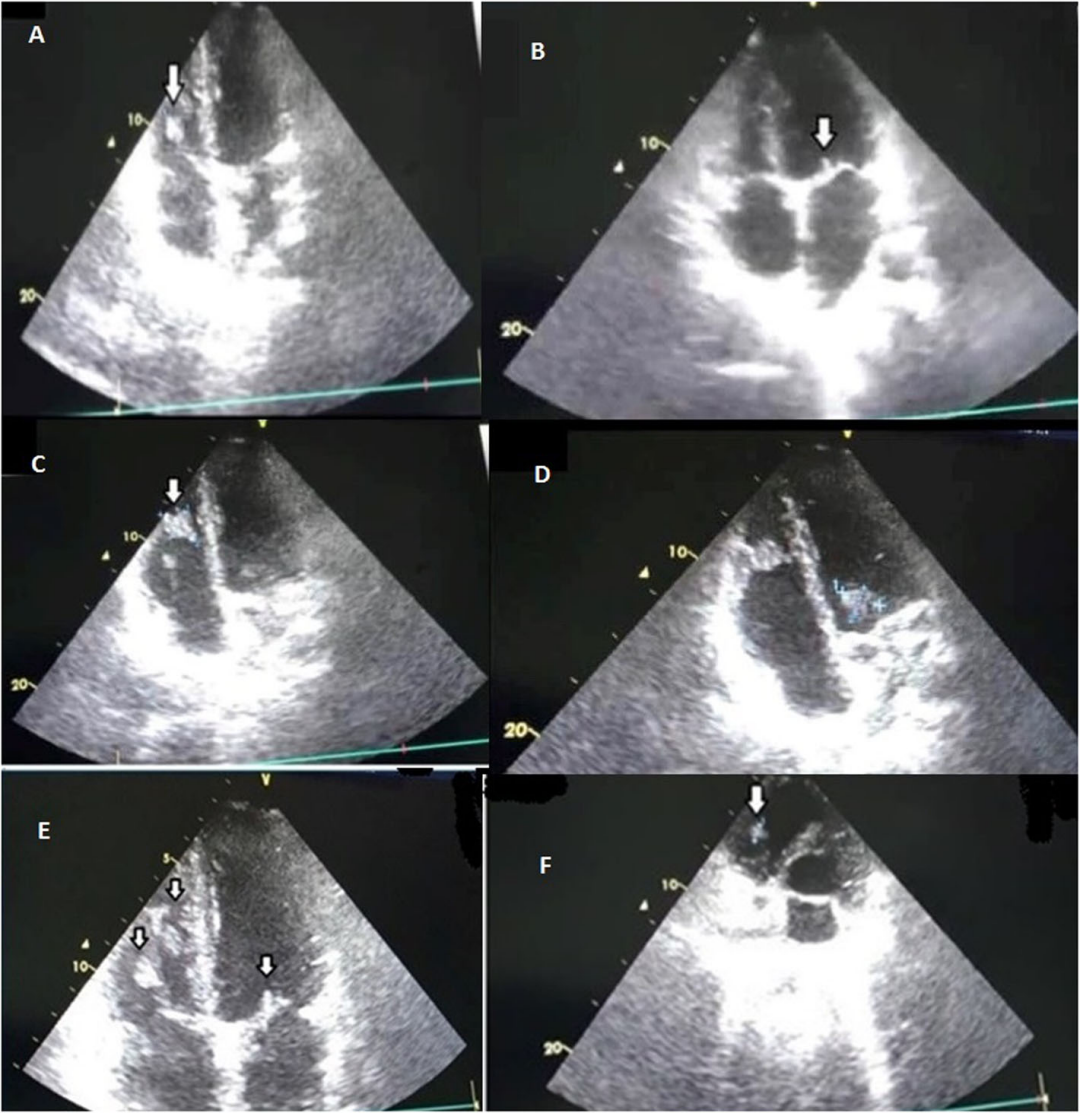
Transthoracic echocardiography (TTE): a four-chamber view showing a large-sized vegetation (arrow) seen on the right ventricular side of the leaflet of the tricuspid valve (TV); b four-chamber view showing a vegetation (arrow) on the ventricular side of the anterior leaflet of the mitral valve; c four-chamber view showing a large vegetation (arrow) on the lateral wall of the right ventricle; d Parasternal long axis view showing two large vegetations on mitral valve and lateral wall of RV; e Parasternal long axis view showing three large vegetations on mitral and tricuspid valves and lateral wall of RV; f Parasternal short-axis view showing a large vegetation on the ventricular side of the leaflet of TV
